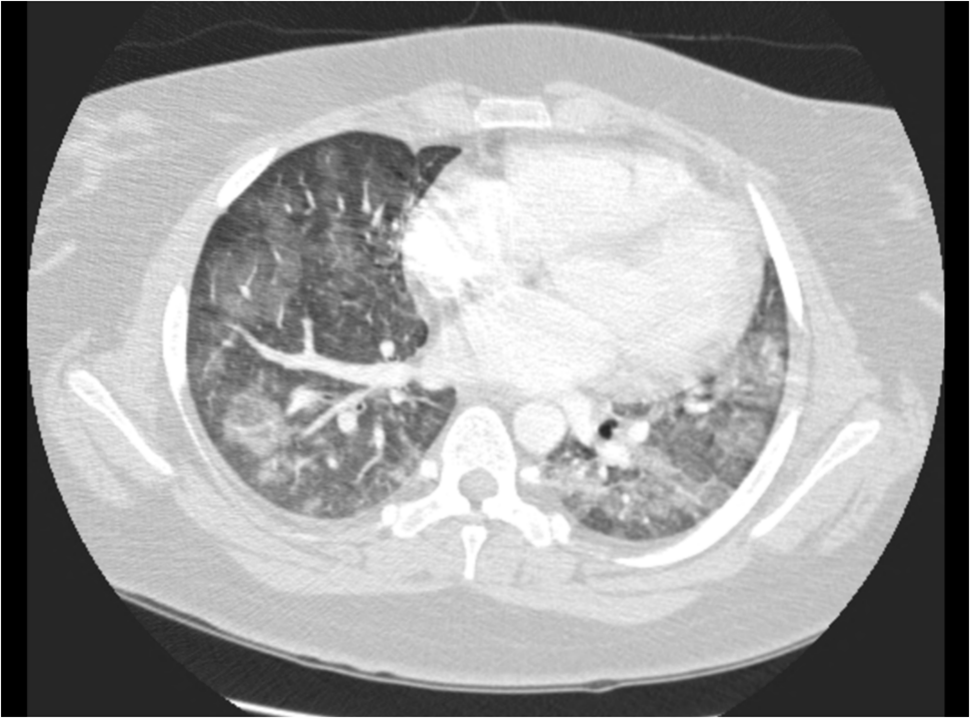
18-year-old female diagnosed with systemic lupus erythematosus when she was 16 years old presenting acutely. Axial CT image with intravenous contrast showing extensive bilateral ground-glass opacity and consolidation suggesting acute lupus pneumonitis
